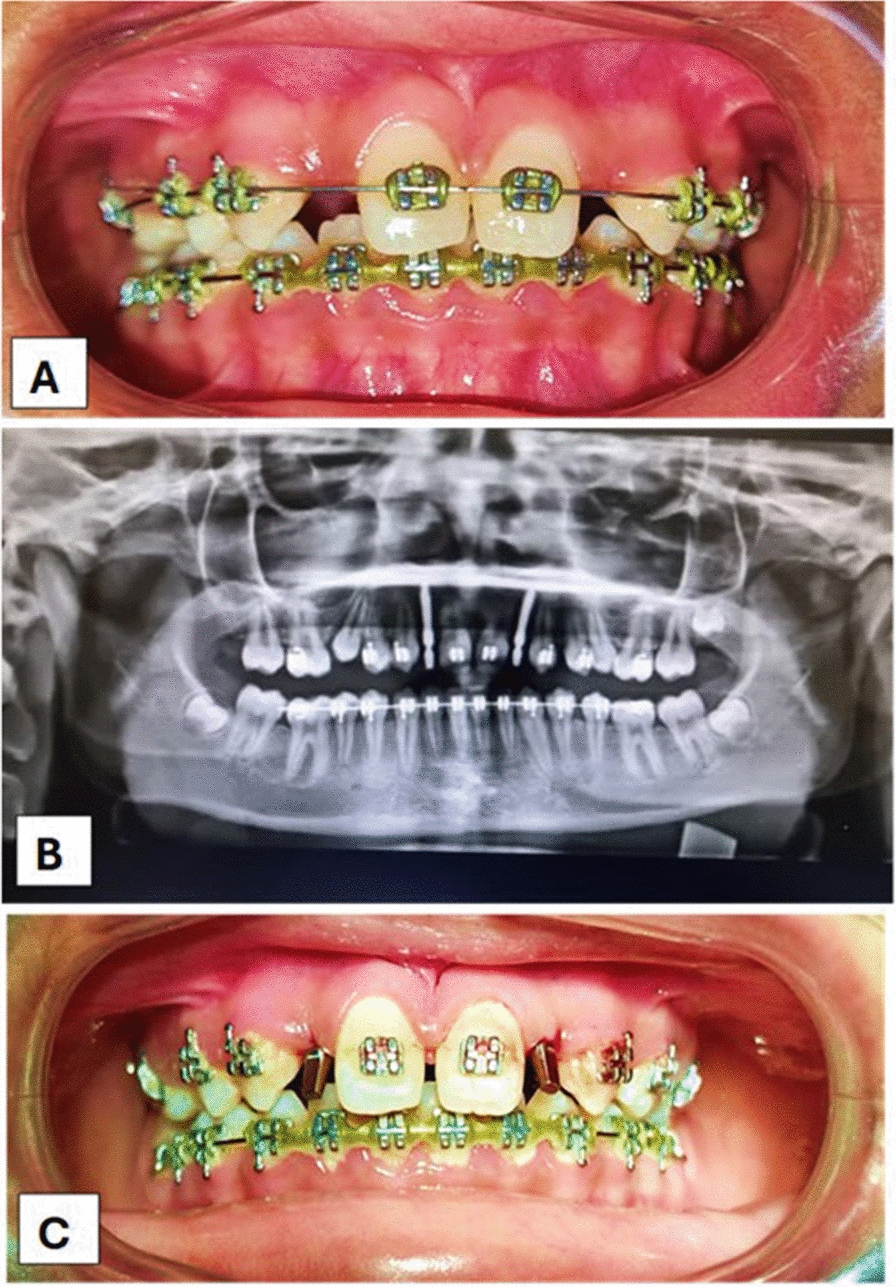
Intraoperative images. A Orthodontic aligning before surgery. B Orthopantomograph after transitional implant placement with 12 and 22. C T = 0, intraoral image of implant abutment immediately postoperatively
